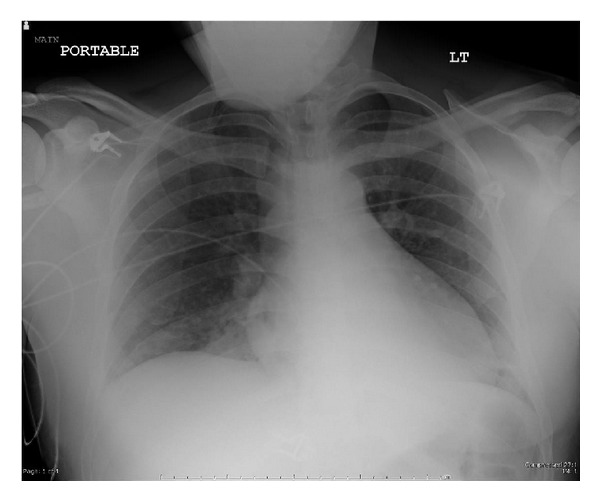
Right lower lung infiltrate on chest X-ray.
